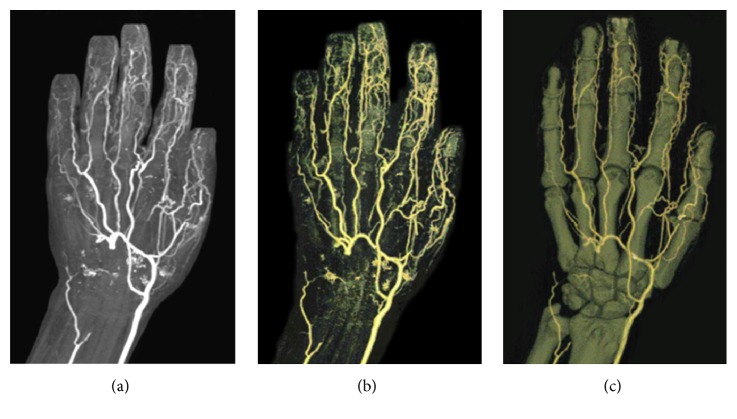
CT angiography of the right upper arm and tridimensional reconstructions ((a)–(c)). The follow-up three months later at the end of an intravenous iloprost course revealed a distal revascularization of the deep palmar arch and common digital arteries.
